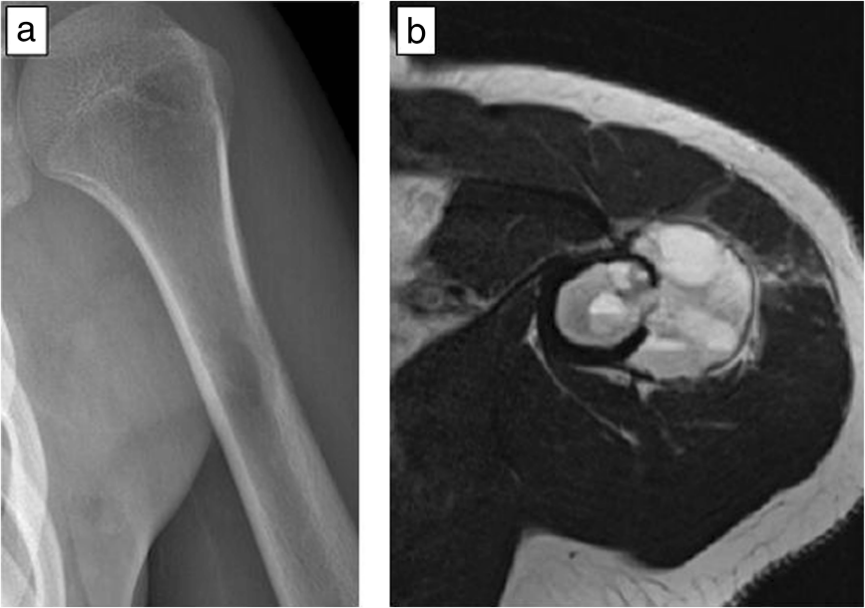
Plain radiograph of the patient showed an osteolytic lesion in the proximal humeral diaphysis (a), whereas magnetic resonance imaging revealed an expansile tumour that occupied the medullary cavity and gave rise to a soft tissue component (b).
